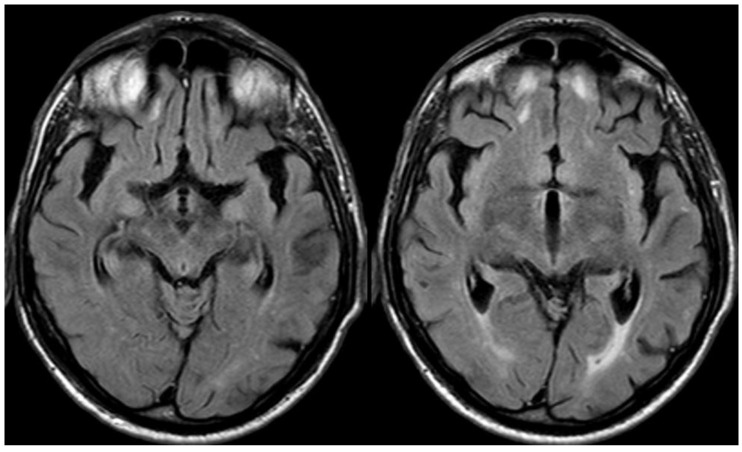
Brain MRI with unenhanced axial FALIR focused on the temporal lobes, showing the resolution of spontaneous sulcal hyperintensity and cortical swelling. The contrast administration did not find further issues.
